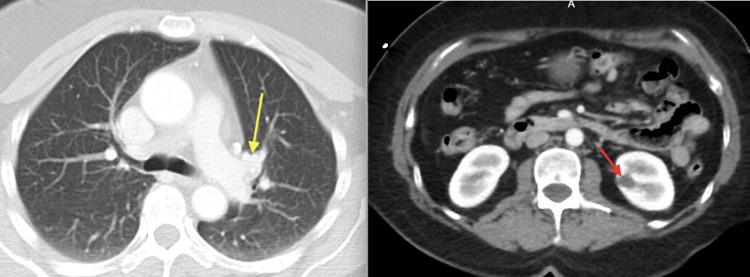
CT of the chest and abdomen. CT of the chest (left) demonstrates old left hilar calcifications (yellow arrow), and CT of the abdomen (right) demonstrates a benign renal cyst (red arrow), with no evidence of malignancy.
